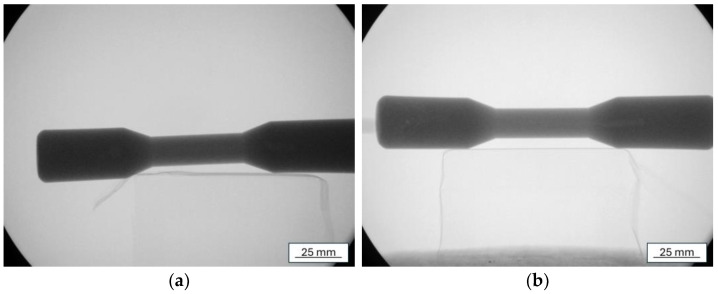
Radiographic examination of castings: trial no. 3 (a) and trial no. 7 (b).
